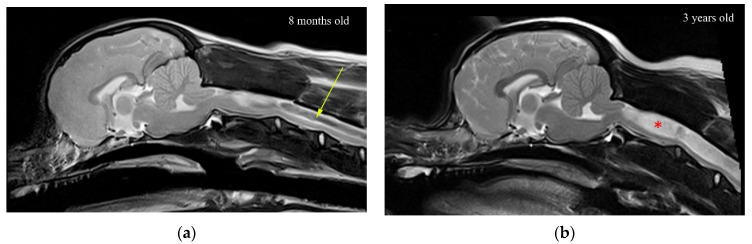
T2-weighted mid-sagittal MRI of the brain and cranial cervical spinal cord in CKCS with Chiari-like malformation and developing syringomyelia: (a) 8 months and (b) 3 years old. In (a), there is a (black) fluid signal-void sign within the center of the developing syrinx suggesting pulsatile or turbulent flow (arrow). In (b), the (white) syrinx has progressed to a large fluid-filled cavity within the spinal cord (red asterisk). There is less (black) fluid void sign suggesting less turbulent flow in this established syrinx.
